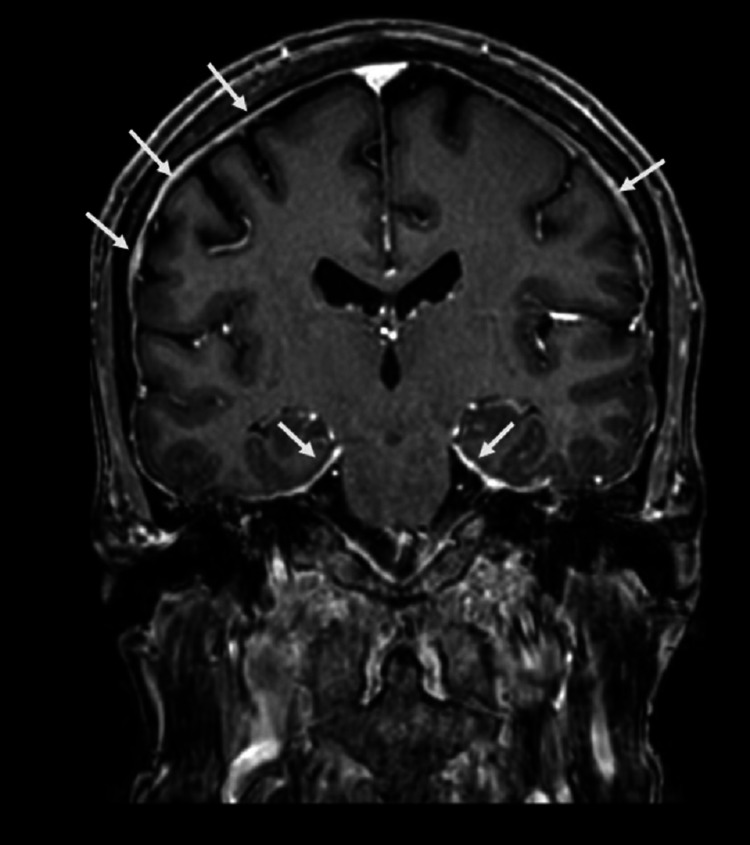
MRI of the brain postcontrast T1 showing diffuse dural enhancement
